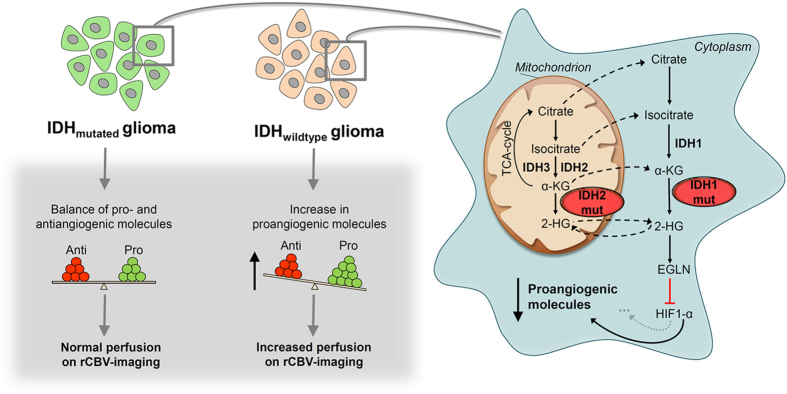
Synopsis of how *IDH* mutation status is linked to angiogenesis and alteration in rCBV on DSC-MRI in human glioma based on this hypothesis generating study. In brief, mutations in cancer-associated *IDH* acquire neoactivity producing 2-hydroxyglutarate *(2HG)*. *(R)-2HG* potentiates *EglN* activity that mark the hypoxia inducible factor *HIF1A* for polyubiquitylation and proteasomal degradation, leading to decreased *HIF1A* activation in *IDH* mutant tumors, compared with their wild-type counterparts. This results in a distinct transcriptome signature induced by upregulation of hypoxia, vasculo- and angiogenesis related signaling pathways in *IDH* wild-type tumors, which is non-invasively detectable with rCBV imaging.
